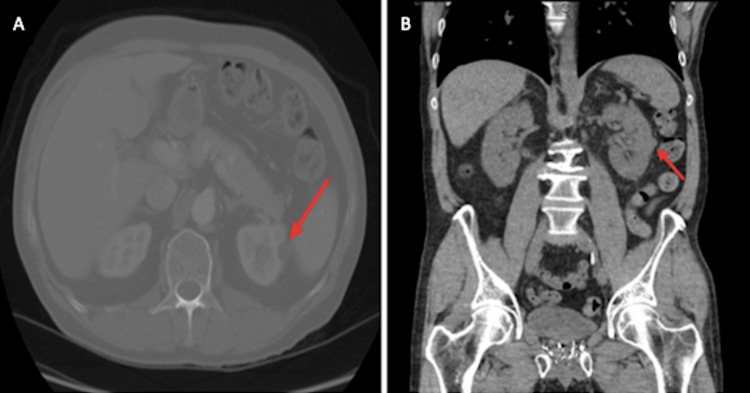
Contrast-enhanced CT abdomen and pelvis CT abdomen in cross-sectional (Panel A) and longitudinal (Panel B) view showed poorly defined solid left renal mass suspicious for renal cell carcinoma (pointed by red arrow).
